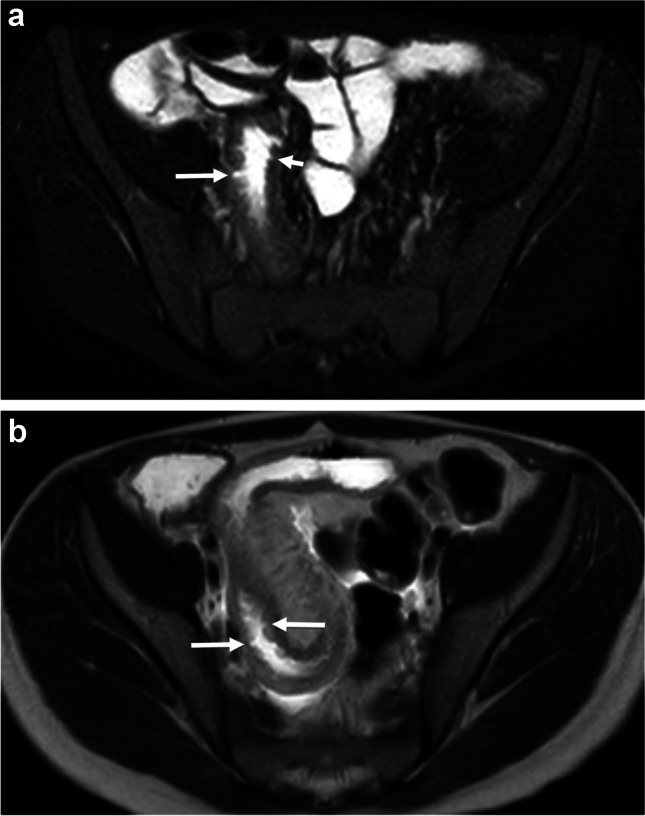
Examples of radiologic ulcers from two different children with newly diagnosed ileal Crohn disease. A Axial T2-weighted single-shot fast spin-echo MR image with fat suppression from a 12-year-old boy shows both deep (*long arrow*) and superficial (*short arrow*) radiologic ulcers. The terminal ileum appears thick-walled with mural edema. B Axial T2-weighted single-shot fast spin-echo image without fat suppression from a different 12-year-old boy shows multiple superficial radiologic ulcers (*arrows*). The terminal ileum appears thick-walled with mural edema. All three study radiologists indicated the presence of radiologic ulcers in these two children as well as agreed on severity (i.e., ulcer depth)
